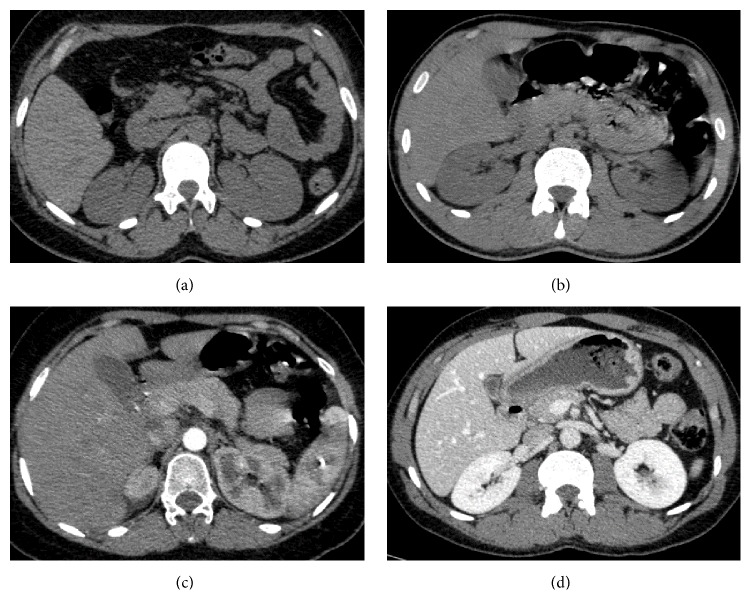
Axial cross-sectional images showing different CT data challenges: (a) low contrast, (b) intensity inhomogeneities, (c) fuzzy boundary, and (d) contrast and anatomy differences.
